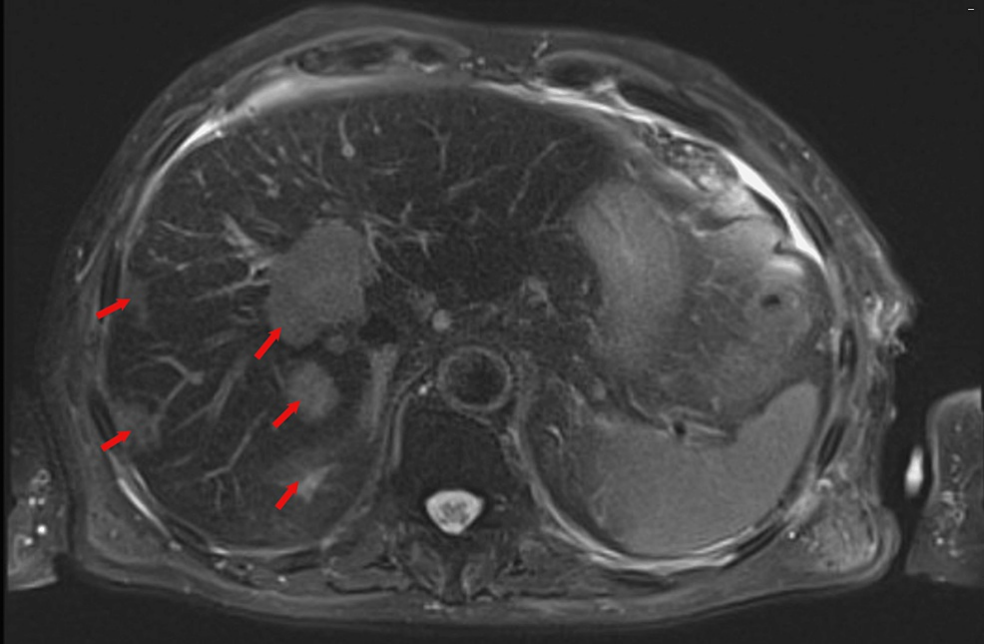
Abdominal magnetic resonance imaging showing the presence of numerous hepatic metastatic nodules (red arrows). The largest one measured 3.5x3x3 cm
